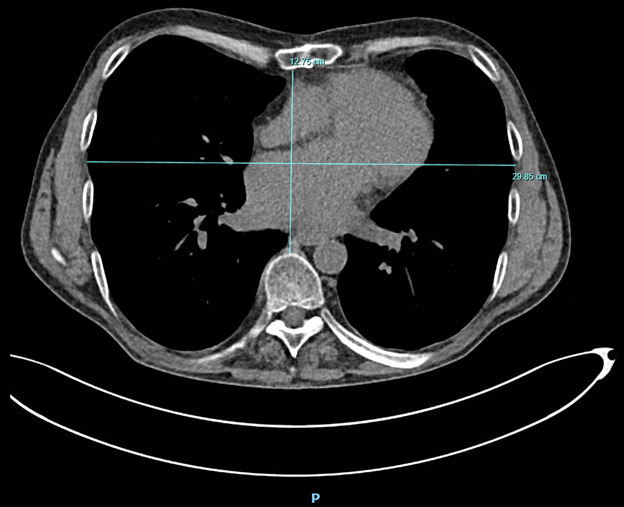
An axial computed tomography (CT) scan of a 58-year-old male
